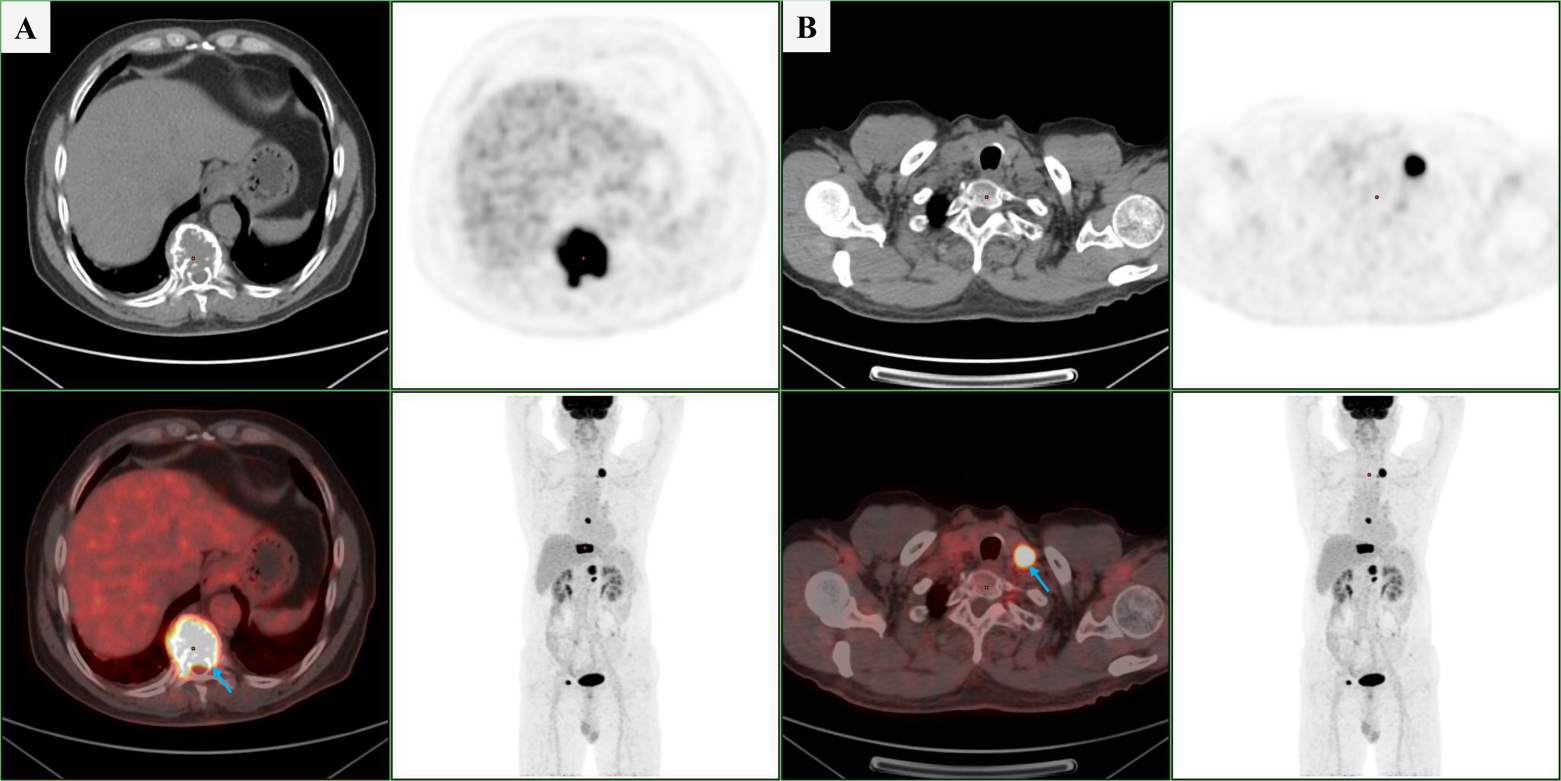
PET/CT demonstrated increased FDG uptake (blue arrow) in the left supraclavicular lymph node (A) and the T10 vertebra (B).
